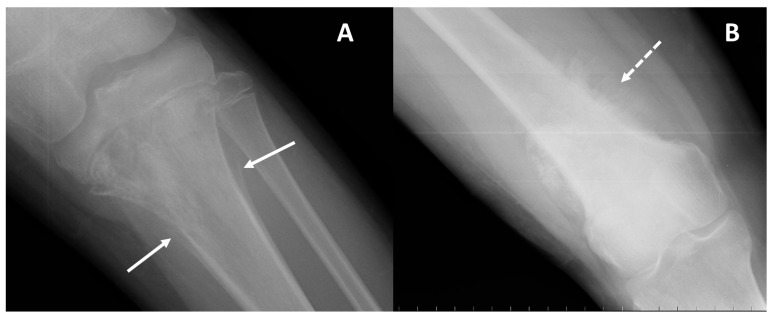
Periosteal reactions encountered on radiographs of osteosarcoma. (A) Conventional radiography of the left knee (AP projection) in an 11-year-old male with osteosarcoma of the proximal tibia presenting as a mixed (mainly lytic) pattern with an aggressive periosteal reaction: the ‘Codman triangle’ type (arrows). (B) Conventional radiography of the right thigh in a 55-year-old female with osteosarcoma of the distal femur presenting as a mixed (mainly eburneus) pattern with an aggressive periosteal reaction: the ‘Sunburst’ type (dotted arrow).
